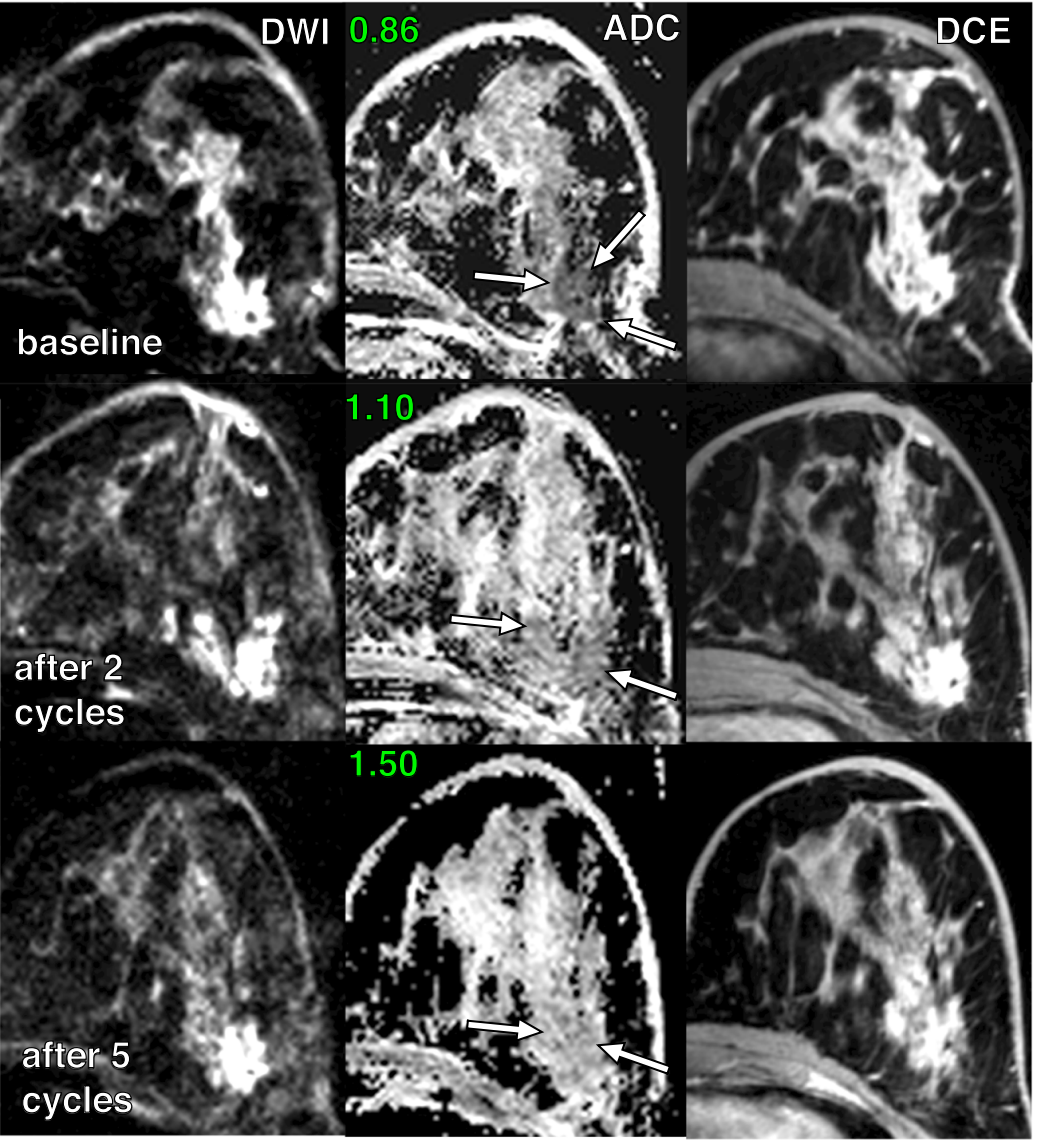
Examples of DWI and CE MRI in a 39-year-old patient with invasive ductal carcinoma and stable disease after neoadjuvant chemotherapy (non-responder). Mean ADC values (×10^-3^ mm^2^/s) measured before and during the therapy are depicted next to the corresponding ADC map, with a region of interest surrounding the lesion (*white arrows*)
